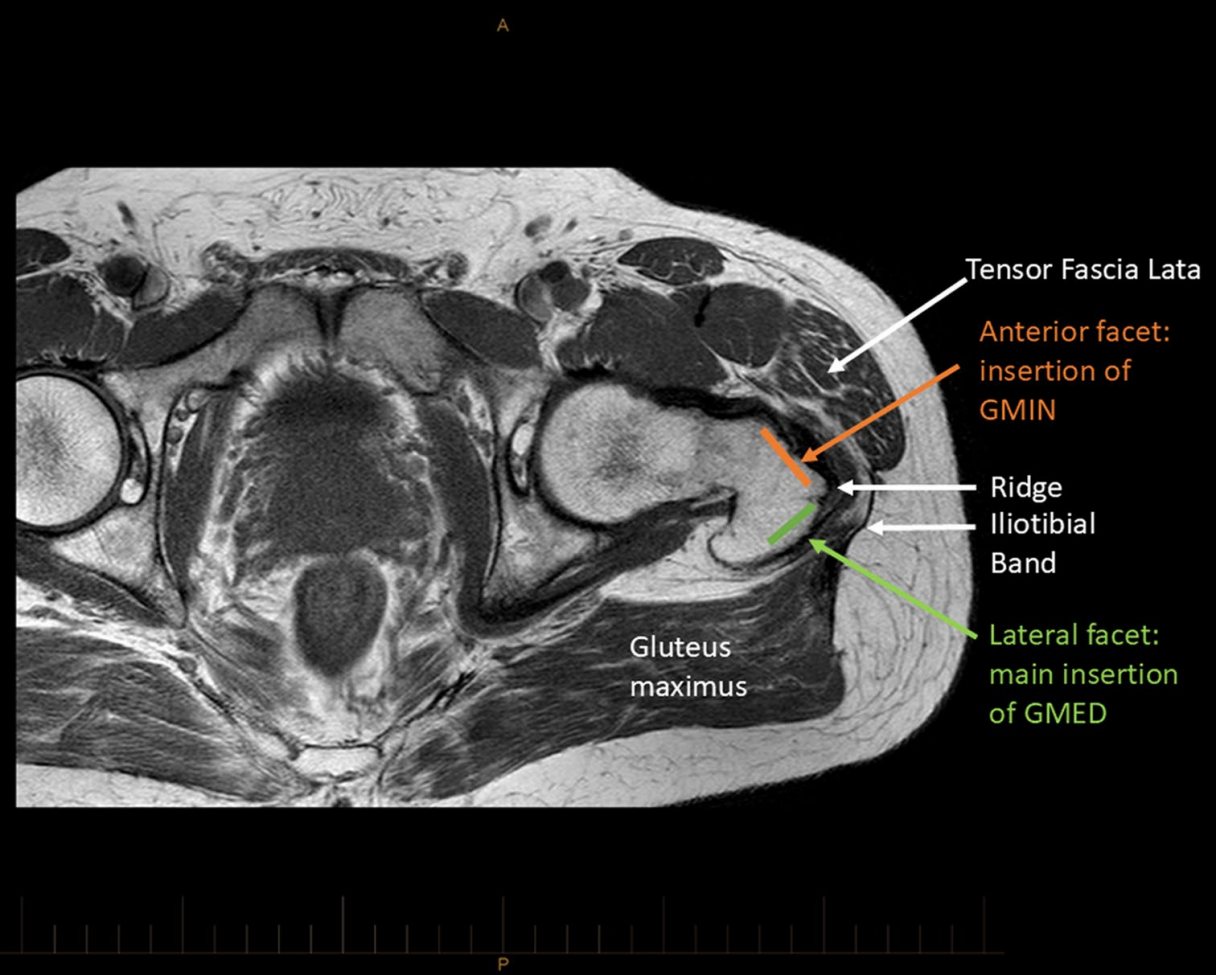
Axial cross-section of a T1 weighted MRI at the level of the greater trochanter, demonstrating the anterior and lateral facets of the greater trochanter, and the overlying iliotibial band and muscular tensioners - tensor fascia lata and gluteus maximus. GMED: Gluteus Medius; GMin: Gluteus Minimus
